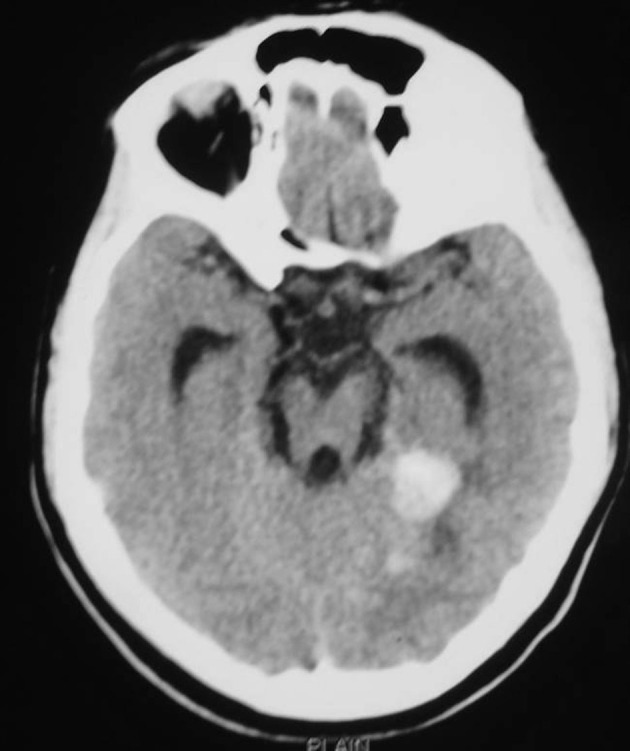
CT scan of the brain showing diffuse subarachnoid hemorrhage and foci of bleed in the right medial posterior temporal region.
